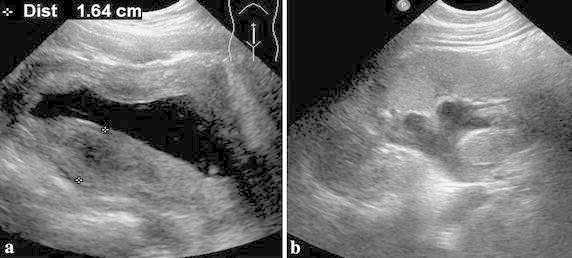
An 8-year-old girl with hemorrhagic cystitis (15th day after hematopoietic stem cell transplantation for aplastic anemia). a Sonography of the bladder shows diffuse type wall thickening of bladder. b Hydronephrosis developed at 23th day after the onset of hemorrhagic cystitis
